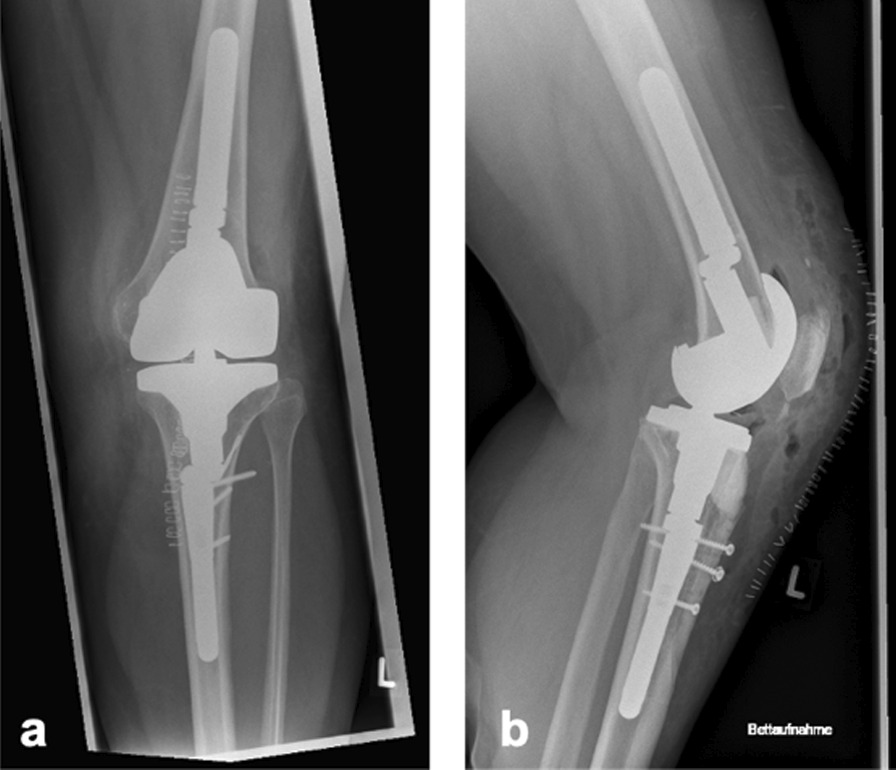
Postoperative x-ray shows cement augmentation of resected tibial tubercle and regular patella position. a Frontal view. b Sagittal view shows cement augmentation and correct patella position without cranial dislocation
